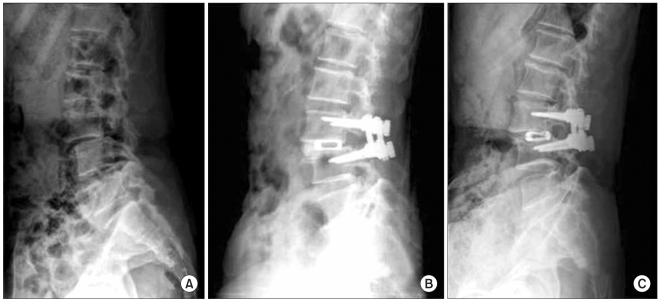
A 53 year-old female who presented with a 1-year history of lower back pain and radiating right leg pain. (A) The lateral radiograph shows spondylolisthesis on L3-4. (B) The lateral view after surgery shows restoration of the disc height. (C) The last follow-up radiograph shows breakage of the cage, but the disc space was maintained and there is probable bone bridging without radiolucency and with stability on the flexion and extension views.
